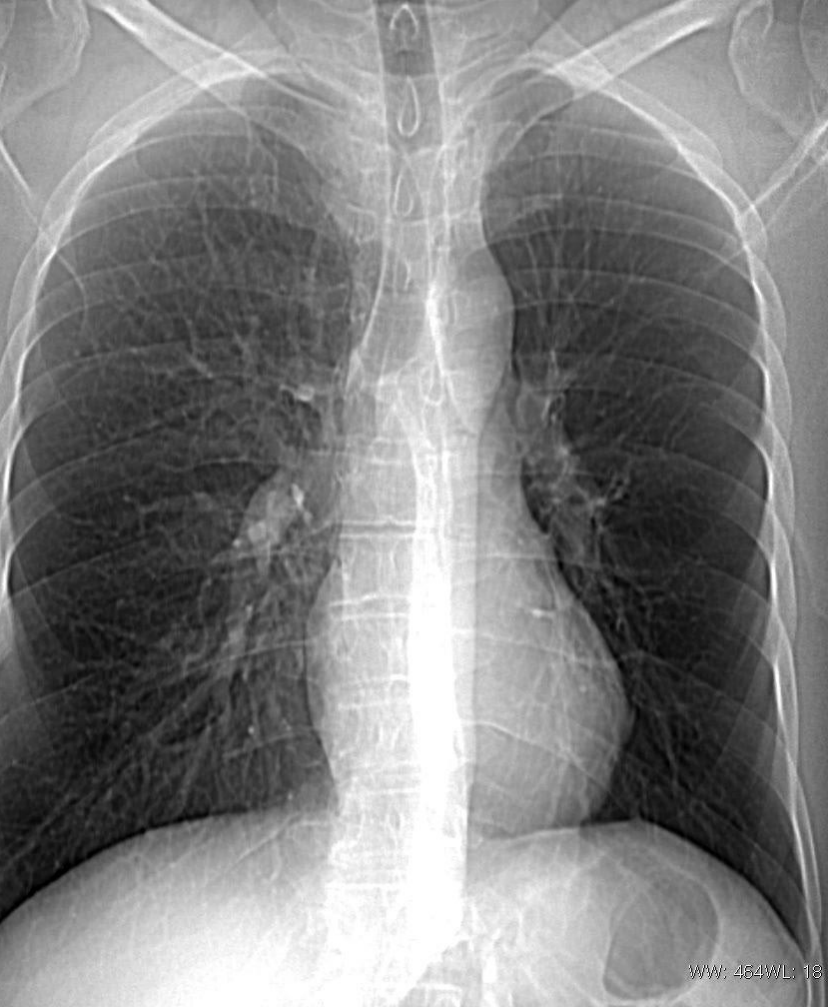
Chest radiograph performed at admission to the hospital.
